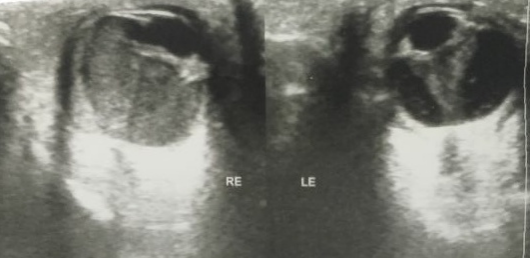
Ultrasound B-scan of the right eye (RE) and the left eye (LE)
